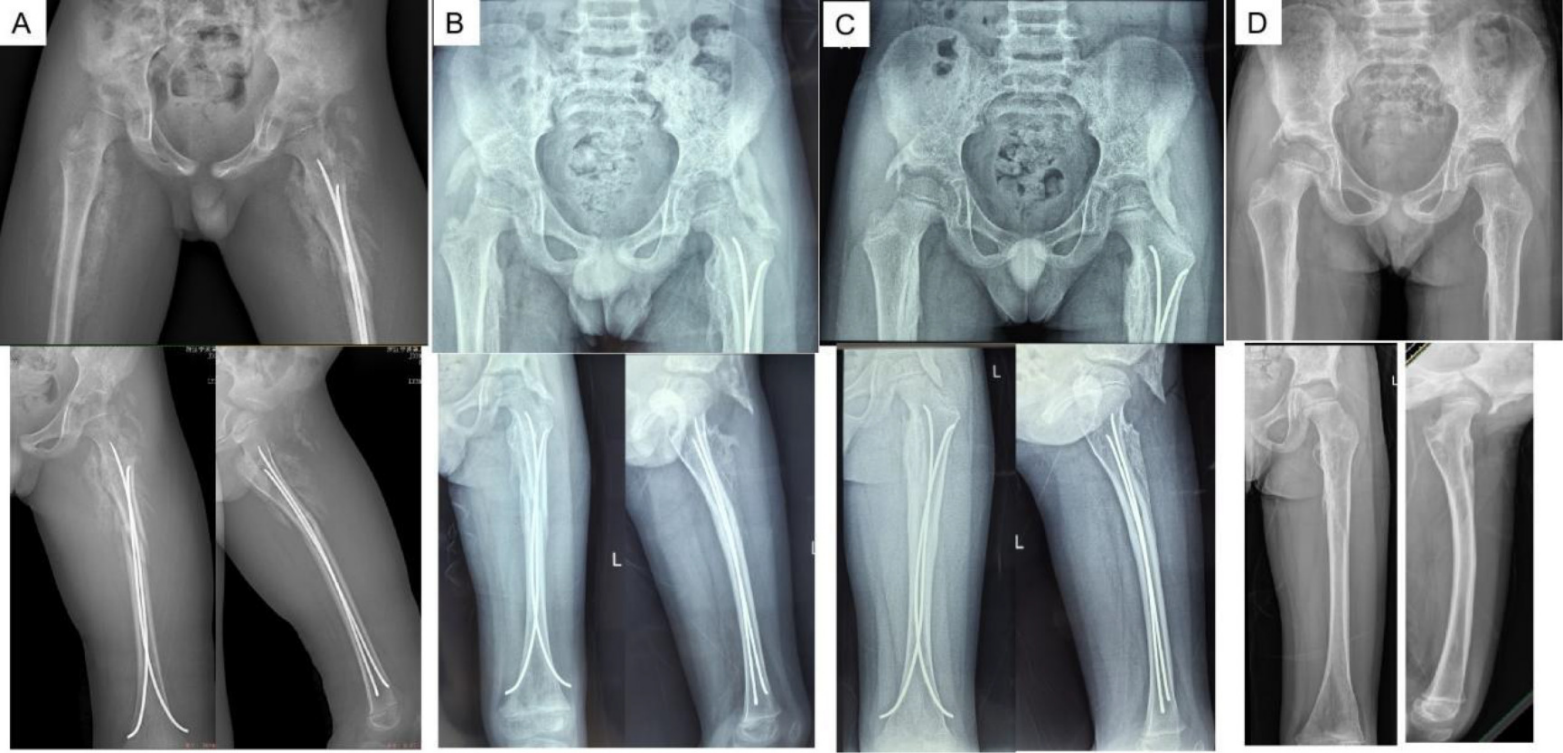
x-rays after surgery [1 month (A), 3 months (B), 6 months (C), 12 months (D)] are shown that the left femoral shaft fracture was healed well and ossising myositis gradually recovered with no signs of recurrence.
